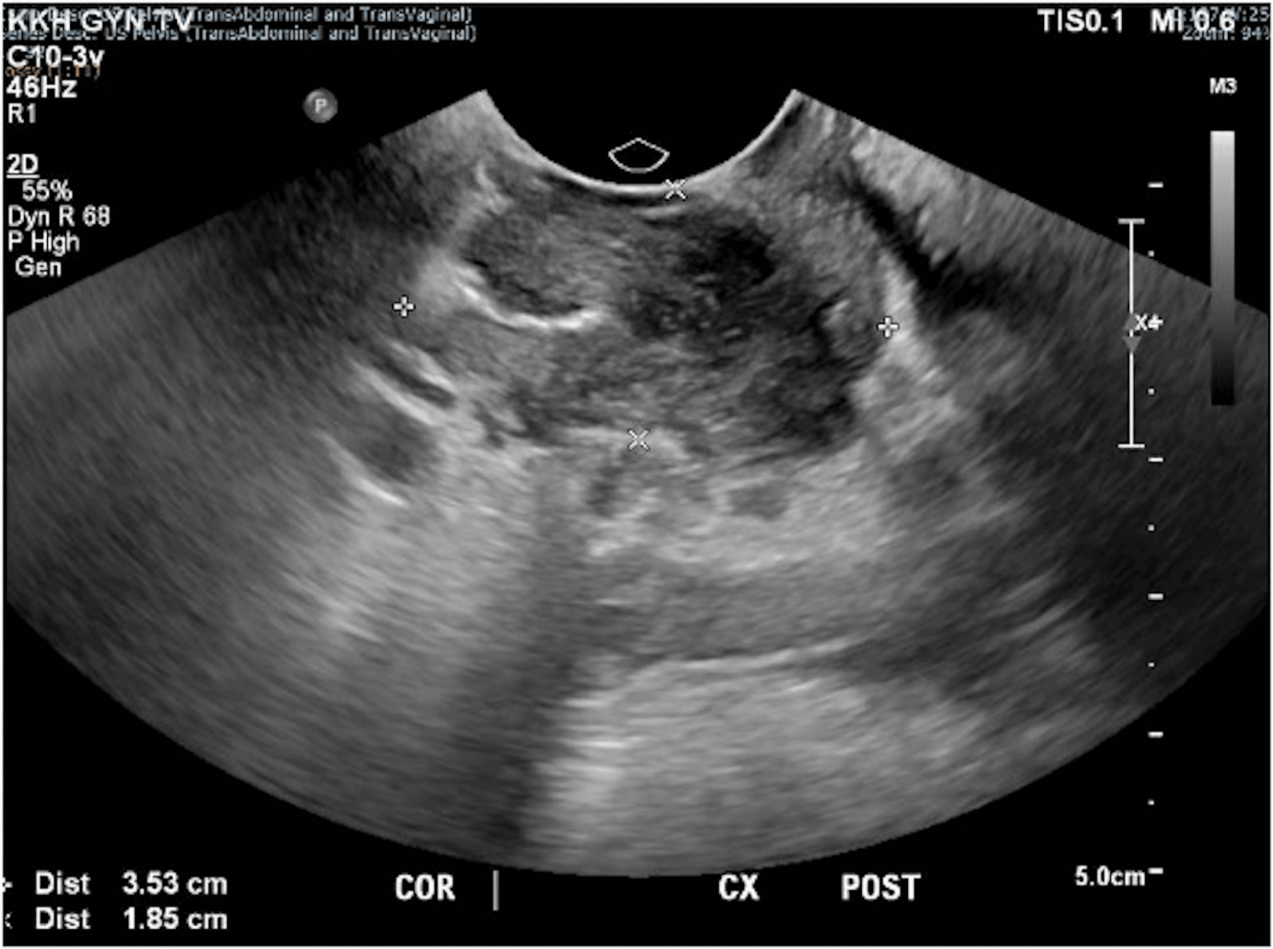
Coronal view of the posterior cervix on transvaginal ultrasonography showing a heterogeneous structure with increased vascularity measuring 3.5 x 2.4 x 1.9 cm posterior to the cervix.
